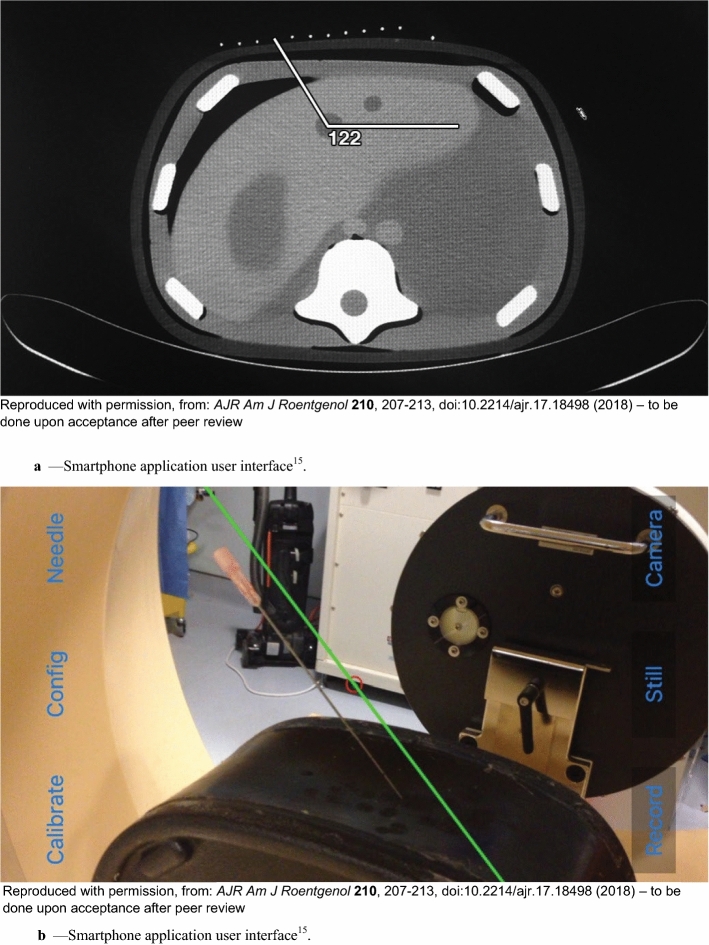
a Smartphone application user interface [15]. a CT image. Lines show planned path on CT console. Number “122” refers to angle of needle in degrees. b Smartphone application user interface [15]. b Screen shot shows interface of mobile application. Green line is guideline that shows planned angle on smartphone's screen. Red circle is center of screen. Buttons are shown in blue. Record button is used to start and Still button is used to stop video recording and image capturing. Camera button is used to switch between front and back cameras. Calibrate button is for registering smartphone to CT scanner. Config, abbreviation meaning “Configure,” button is for setting up wireless connection between smartphone and local PC to allow optional image display on PC and additional control from PC. Needle button is used to set planned needle angle on smartphone
